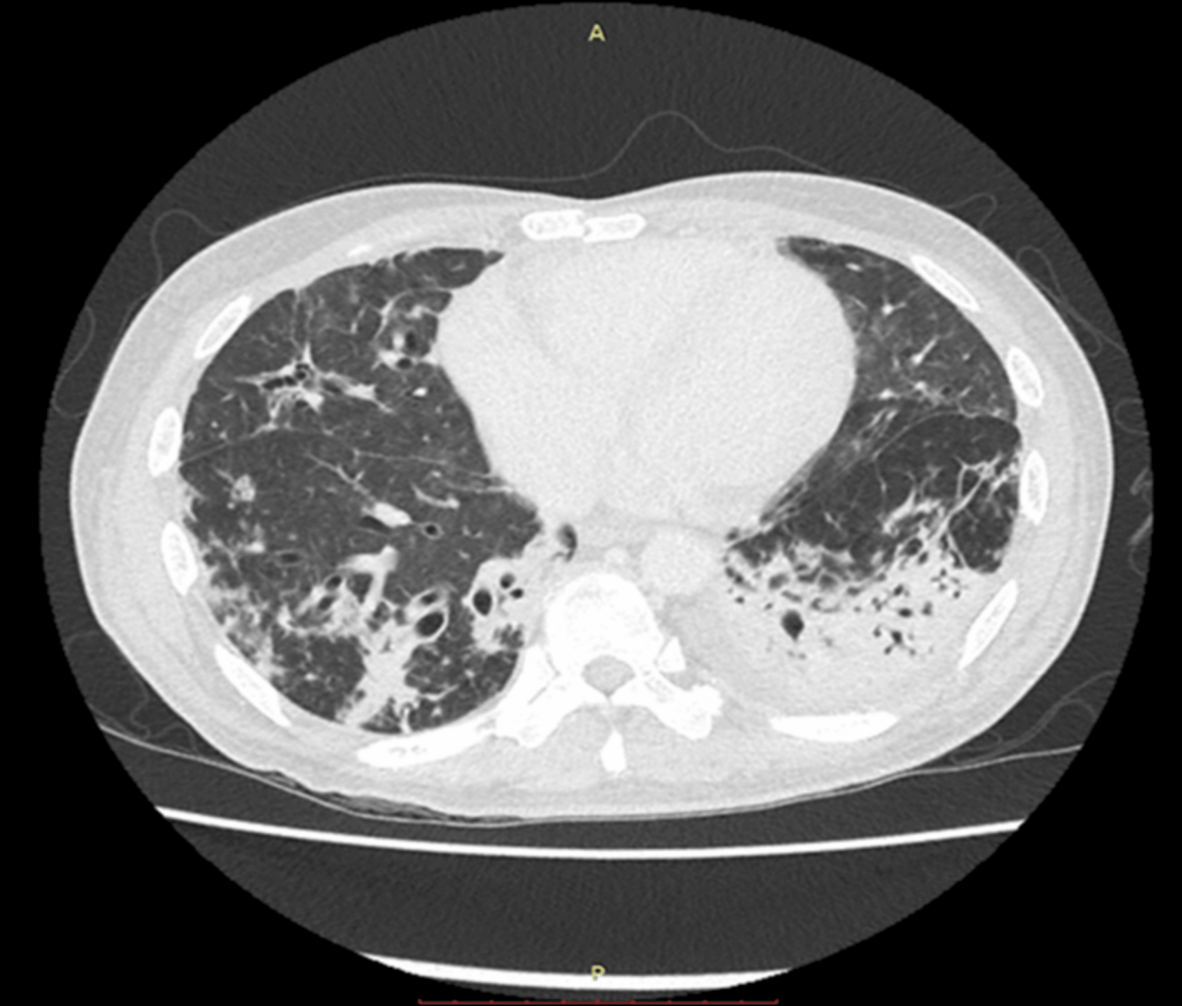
CT scan of the thorax showing consolidation with an air bronchogram in the posterior segment of bilateral lower lobes, as well as secondary organizing pneumonia, fibrosis, and bronchiectasis.
